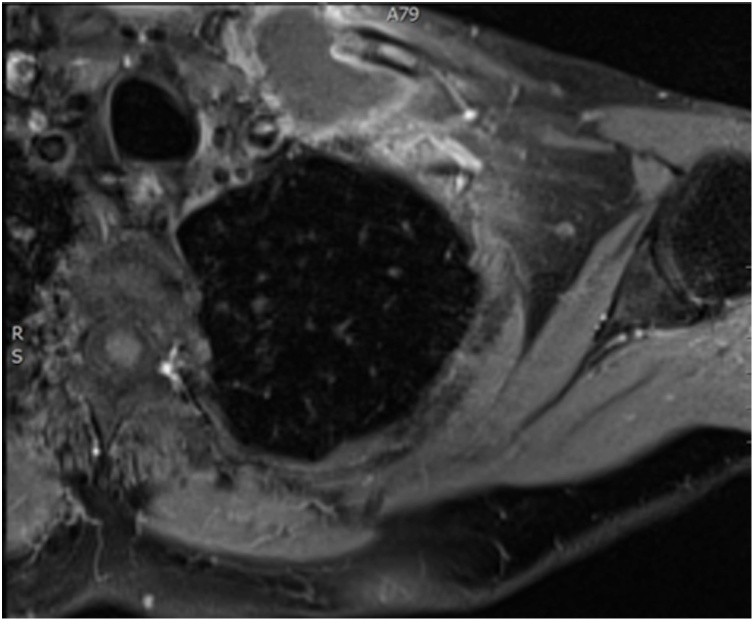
CT of thorax. There is a bilobular low-density mass with an intermediate density wall in the left side of root neck measuring approximately 6.5 × 3 cm in maximum axial dimensions and 4 cm craniocaudially. It abuts the superior aspect of clavicle extending of anterior and posterior to it. The periostal was seen sclerotic in the left clavicle in comparison to the right side. The left subclavian vein and lower left great internal jugular vein appear to be compressed by the mass.
